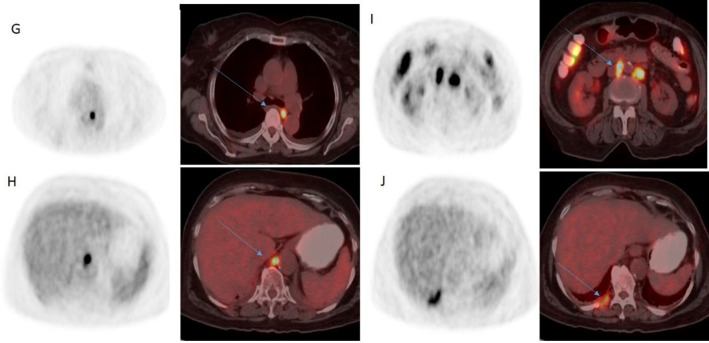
FDG PET (SUV range: 0-4) and PET/CT fused transaxial images revealed FDG avid mediastinal (G), retrocrural (H) and retroperitoneal (I) lymphnodes and pleural lesion. (J) [Arrow]
